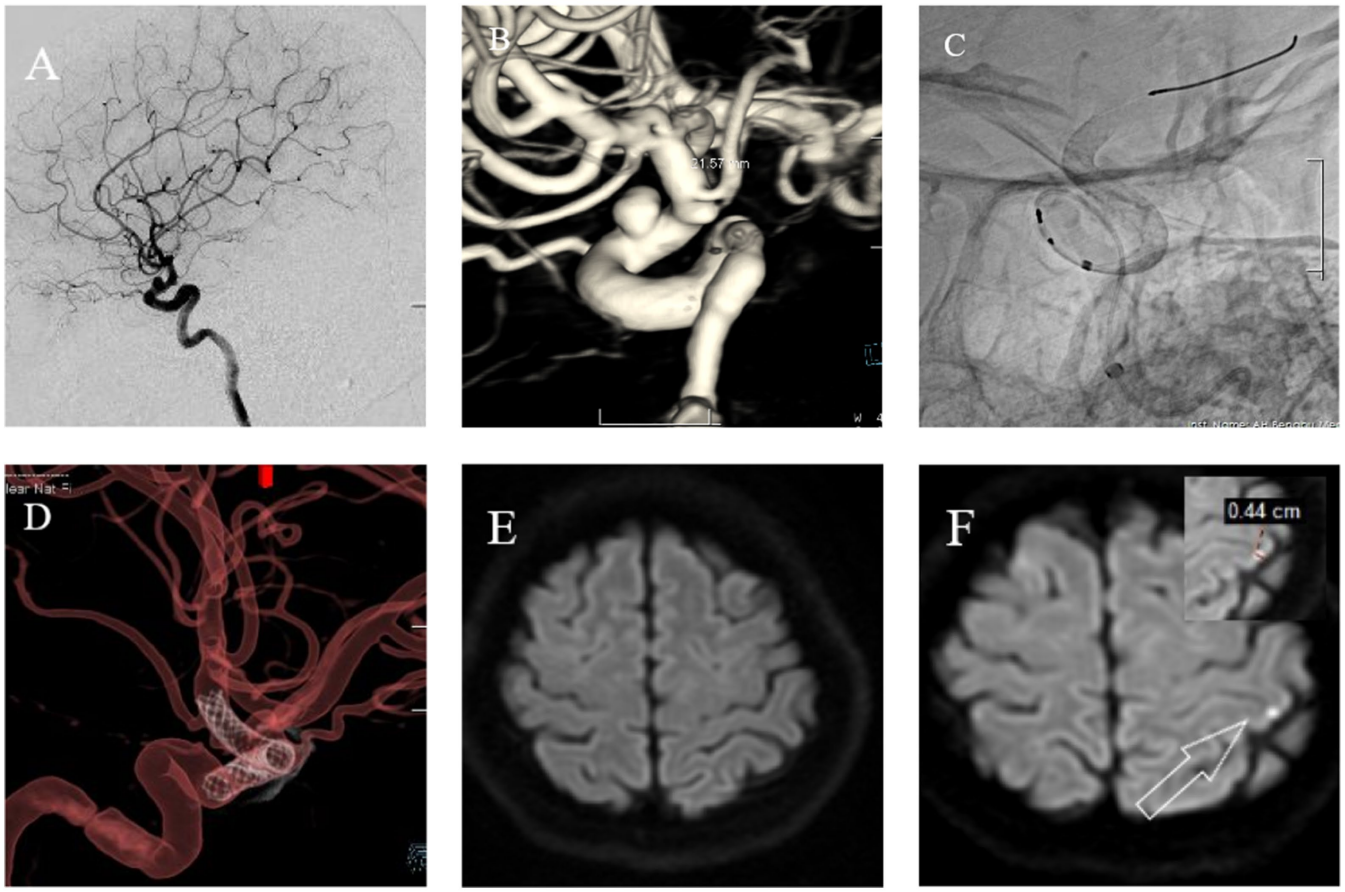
Female, 56 years old. Intermittent headaches for over 7 months, worsening for 1 week. (A) Cranial DSA showing the location of the intracranial aneurysm in the 2D plane during the operation. (B) Cranial DSA showing the location of the aneurysm in the 3D image, confirming it is in the ophthalmic artery segment of the left internal carotid artery. (C) Intraoperative 2D plane showing the location of the stent. (D) Postoperative 3D plane showing the stent model. (E) Preoperative MRI-DWI showed no new infarcts. (F) Postoperative day 3 MRI-DWI showed an acute microinfarction lesion in the left parietal lobe with a diameter of approximately 0.44 cm.
